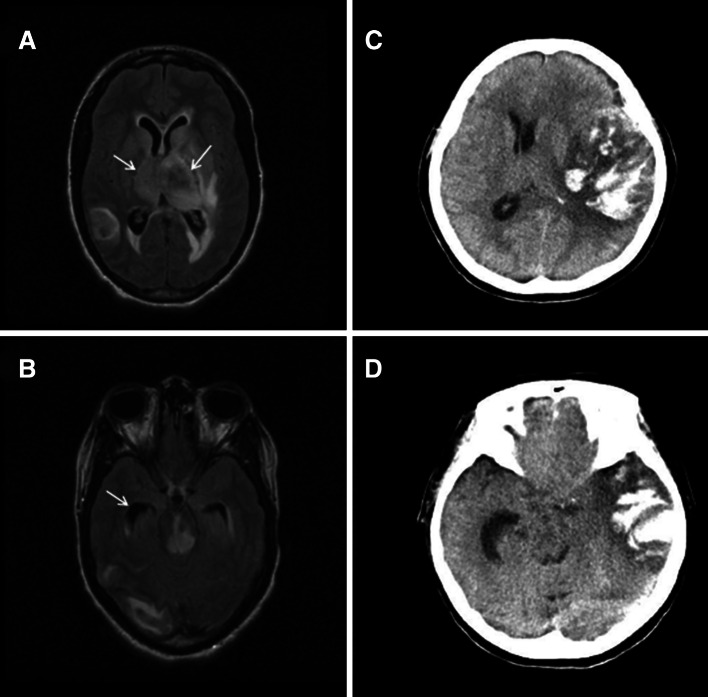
Cerebral imaging of two patients with hydrocephalus. a, b Axial Fluid Attenuated Inversion Recovery (FLAIR) MRI showing extensive edema in the thalami, basal ganglia and brainstem, and hydrocephalus of both temporal horns (*arrows*). c, d Axial non-contrast-enhanced CT scan of a different patient showing a large space occupying intracranial hemorrhage in the left hemisphere, and an increase of width of the contralateral temporal horn
